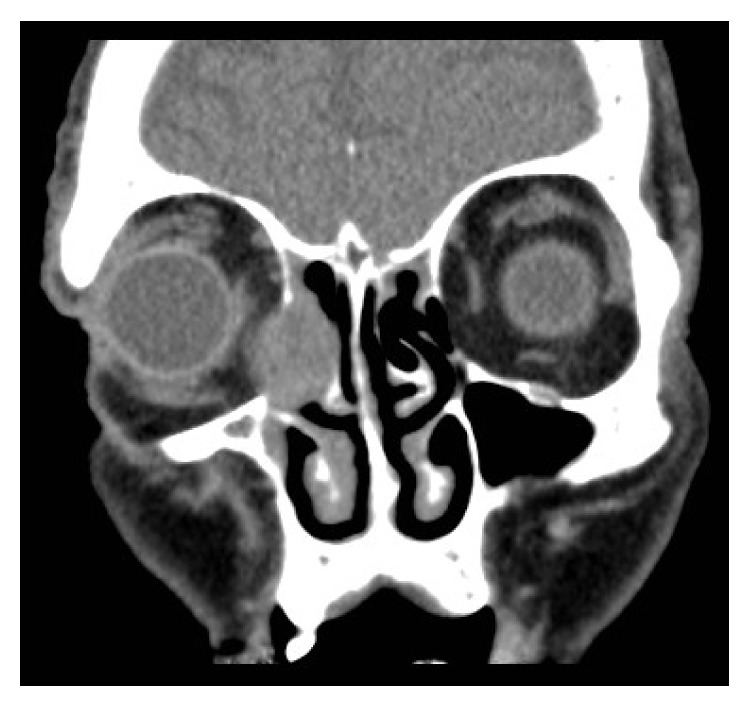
CT orbits: coronal section showing a well-defined heterogeneous lesion protruding into the middle meatus and right orbit.
